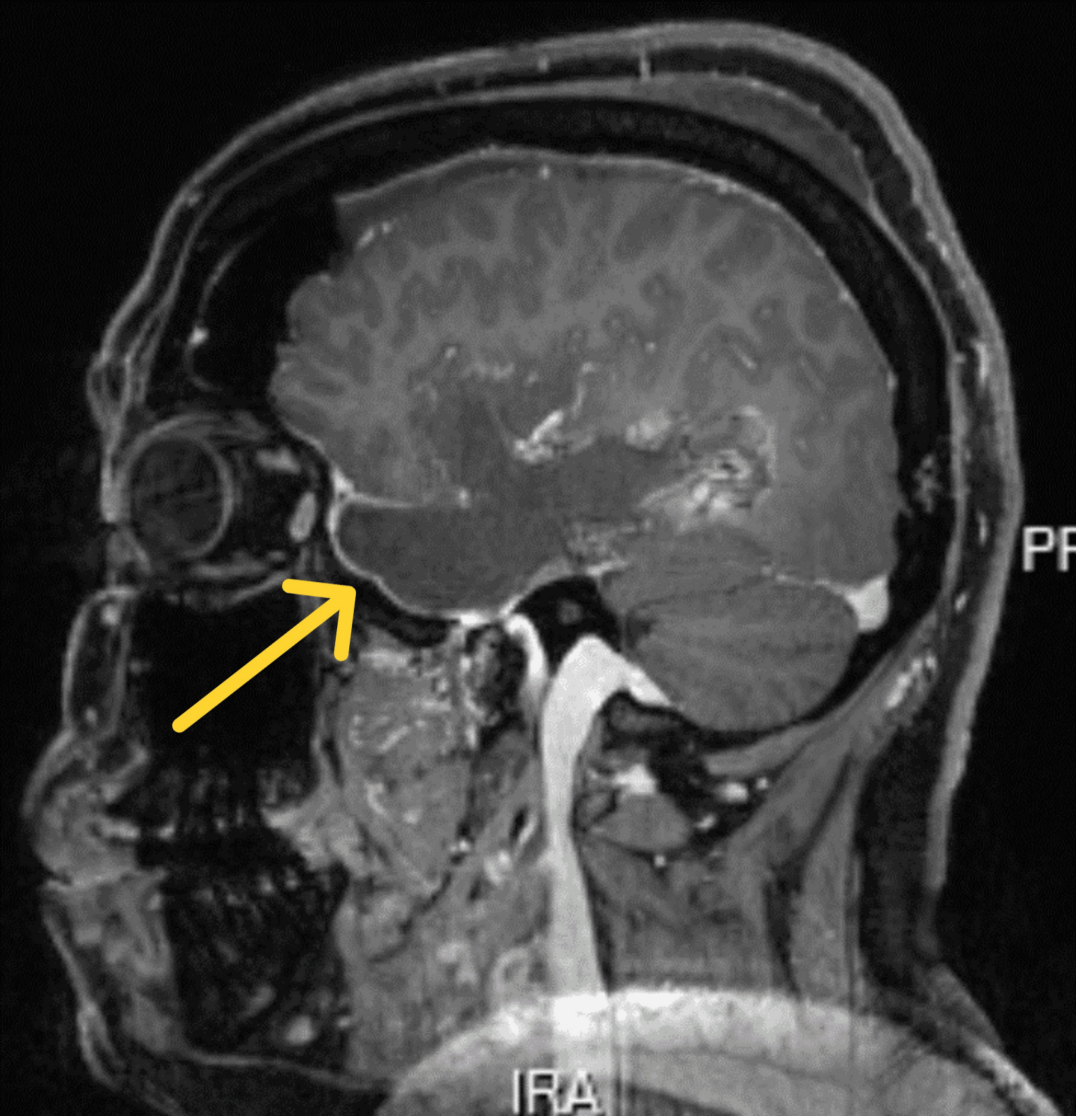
Postoperative MRI (T1 with contrast, axial view) following redo craniotomy and tumor resection.
